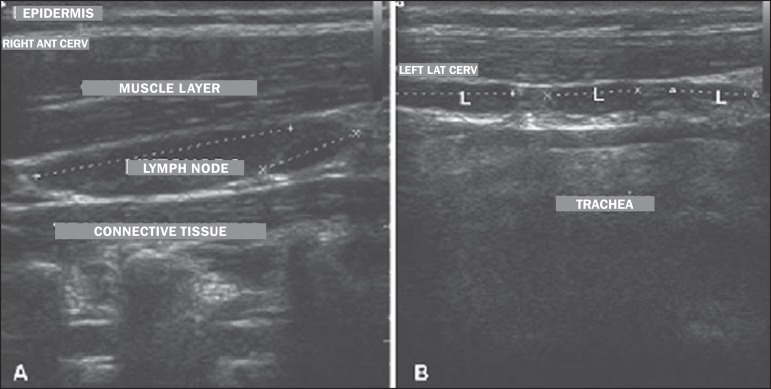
Ultrasound of female subjects (A) and male subjects
